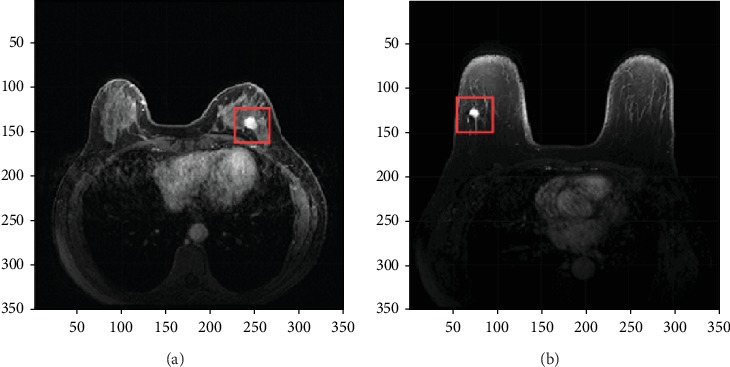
Enhanced sequence MR images: (a) slice of a grade II IDC and (b) slice of a grade III IDC. The red rectangular areas represent the lesion ROI.
